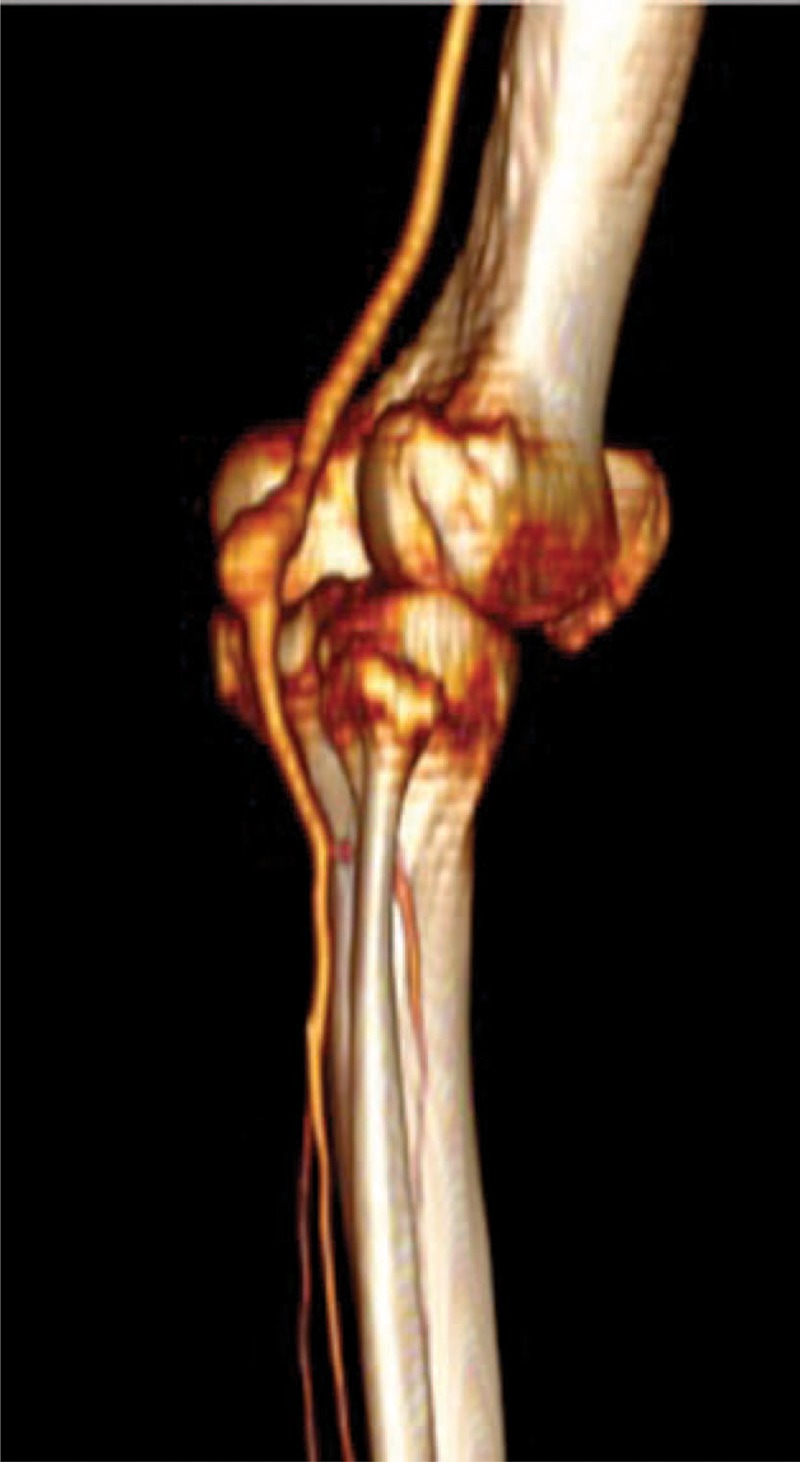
Preoperative CTA angiography three-dimensional reconstruction showing popliteal artey aneurysm location. CTA = computed tomography angiography.
